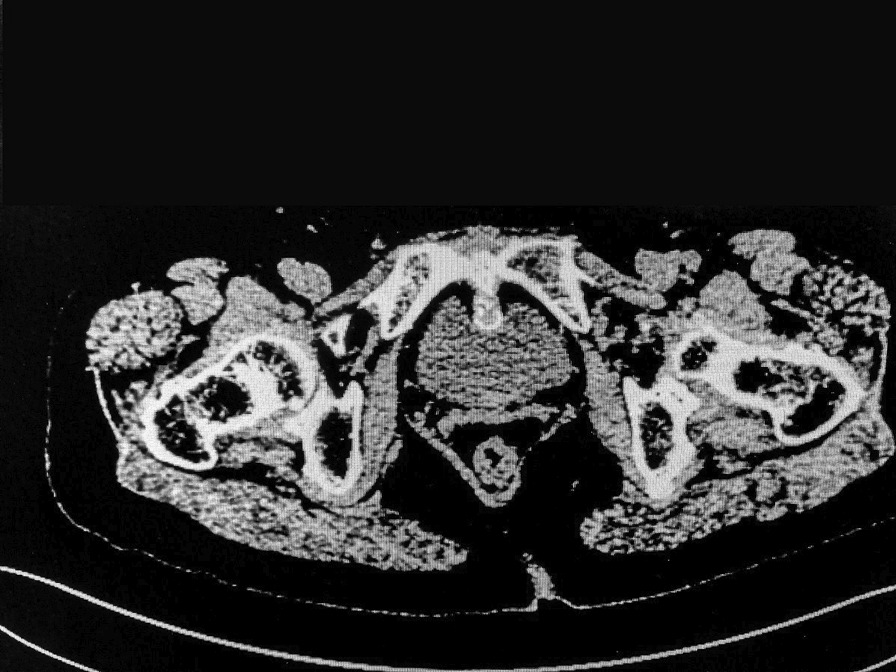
CT showing abnormal changes at the back of the pubic symphysis and compression of the adjacent anterior bladder wall
